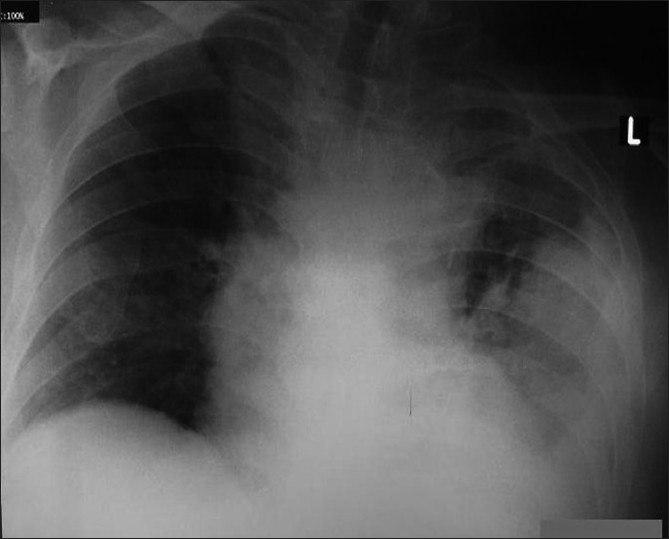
Chest X-ray of the case 2. left-sided large pleural effusion after the VPLS.
